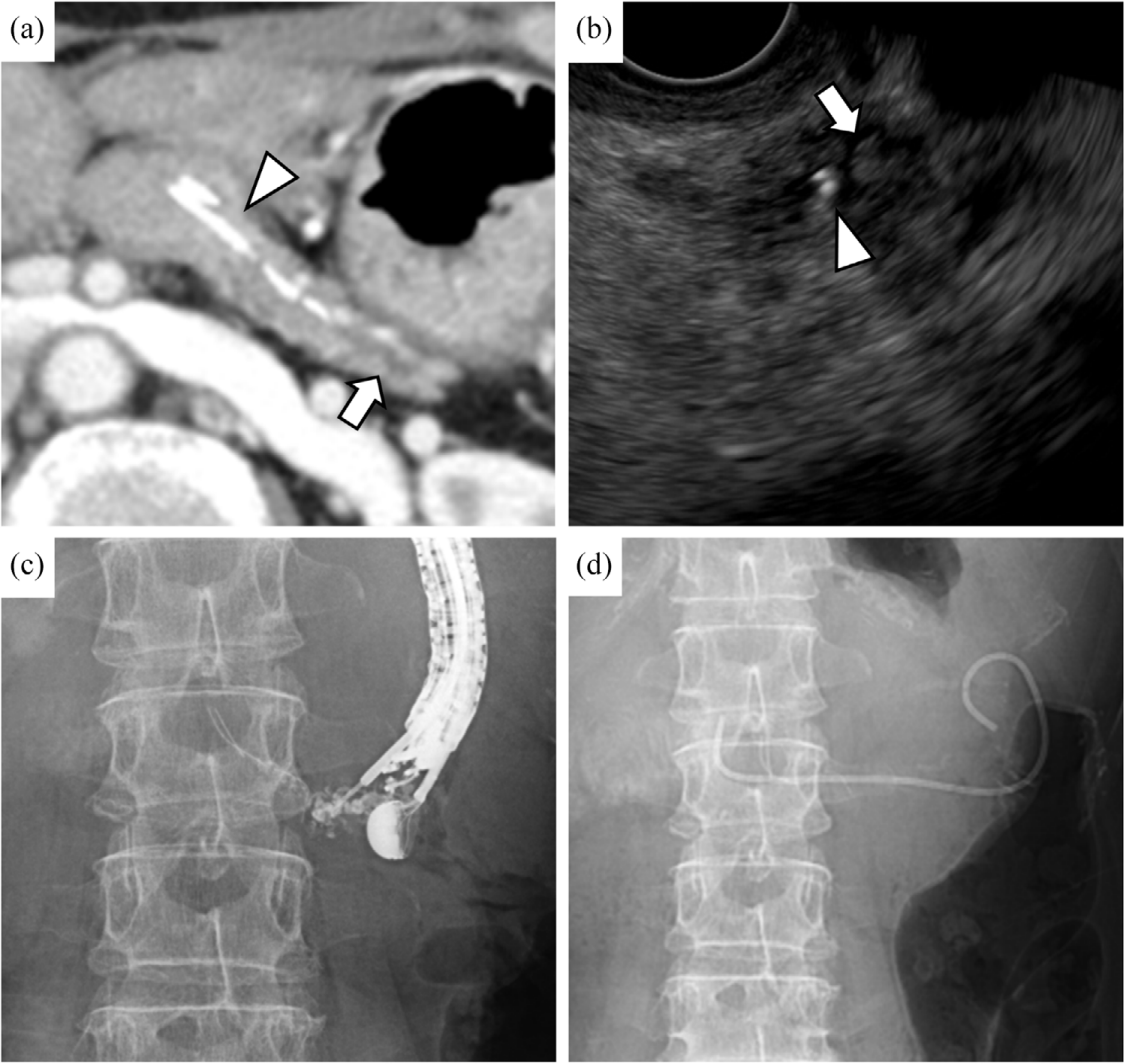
Radiographs showing a stent that had migrated into the pancreatic duct. (a) Using contrast‐enhanced computed tomography, an internal stent (arrowhead) was observed within the main pancreatic duct, along with dilation of the tail side of the main pancreatic duct (arrow). (b) Employing endoscopic ultrasonography, an internal stent (arrowhead) and debris echoes (arrow) were identified within the pancreatic duct. (c) The pancreatic duct was punctured transgastrically using a 19G needle for fine‐needle aspiration. (d) A 6Fr double pigtail plastic stent was placed with one pigtail positioned in the jejunum and the other in the stomach.
